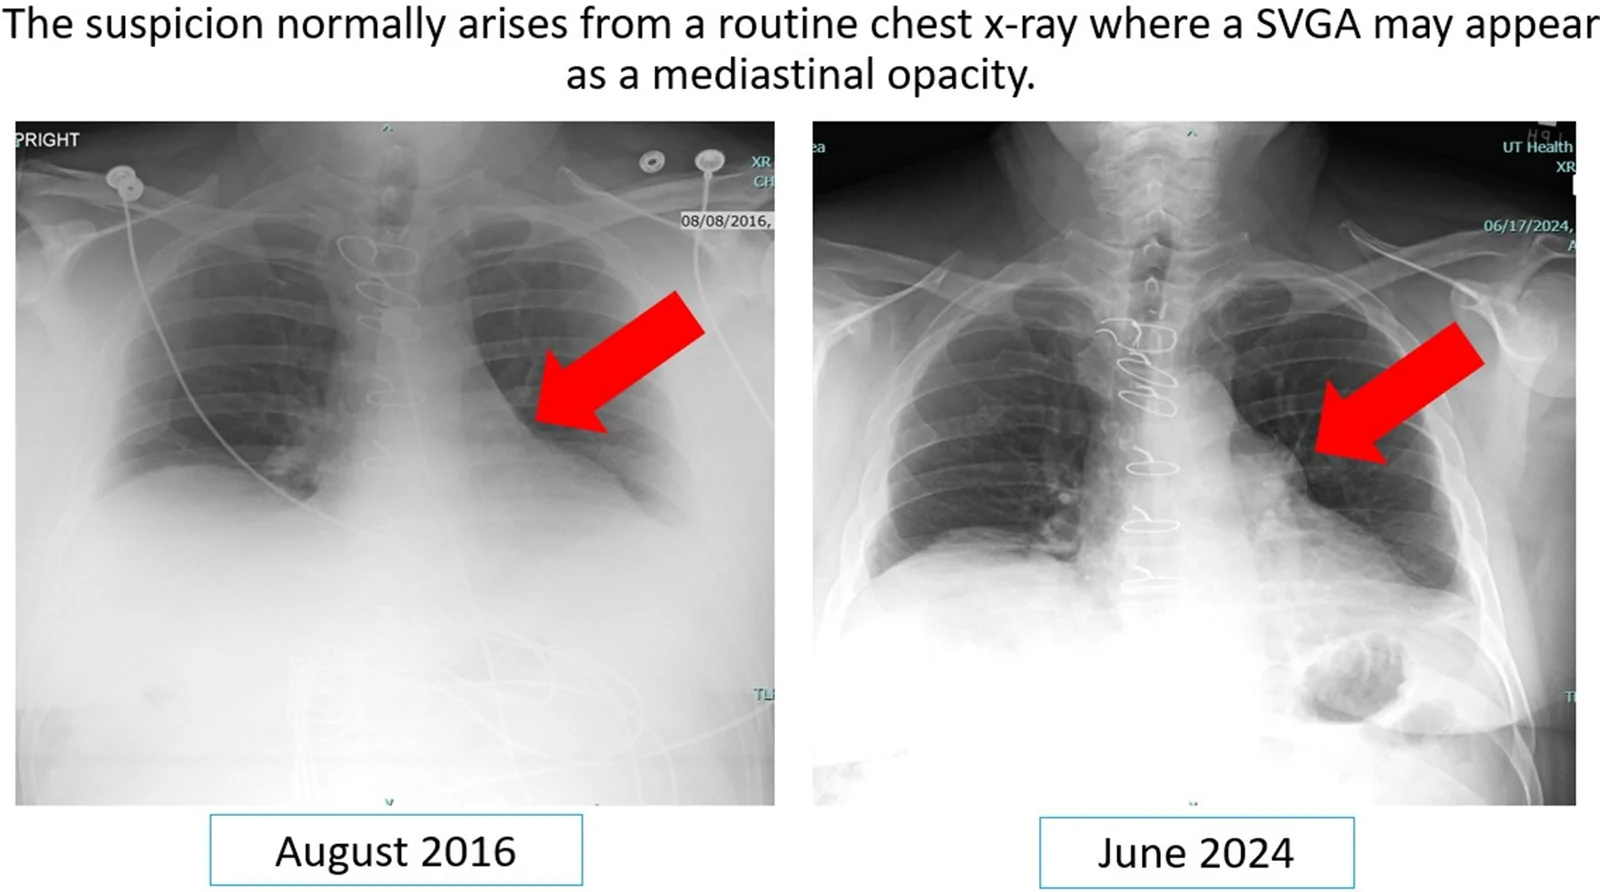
Suspicion of an SVGA May Arise From A Routine Chest X-Ray Where an SVGA May Appear as a Mediastinal Opacity Red arrows demonstrate the interval progression of the mediastinal opacity. SVGA = saphenous vein graft aneurysm.
